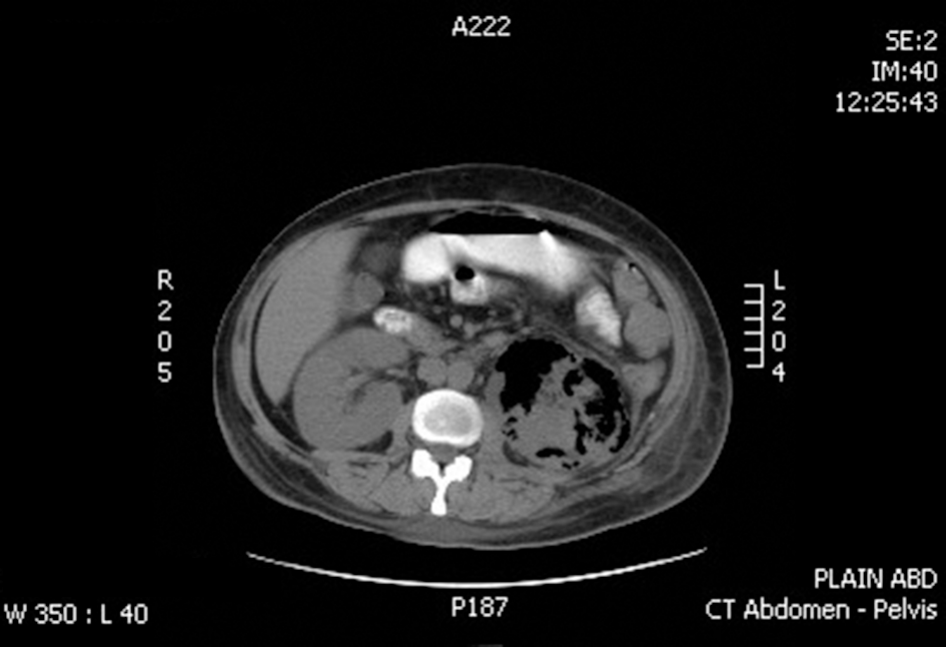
CT Features of Left Sided Emphysematous Pyelonephritis (Axial Cut)
